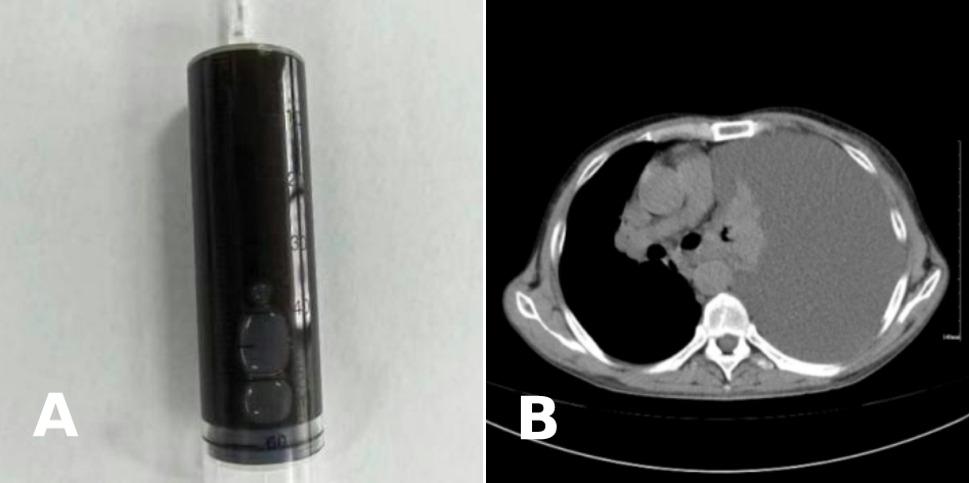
(A) Black pleural fluid obtained via thoracic drainage from the left pleural cavity. (B) Axial chest CT image demonstrating massive left-sided pleural effusion with obscuration of the left lung and mediastinal shift to the right
